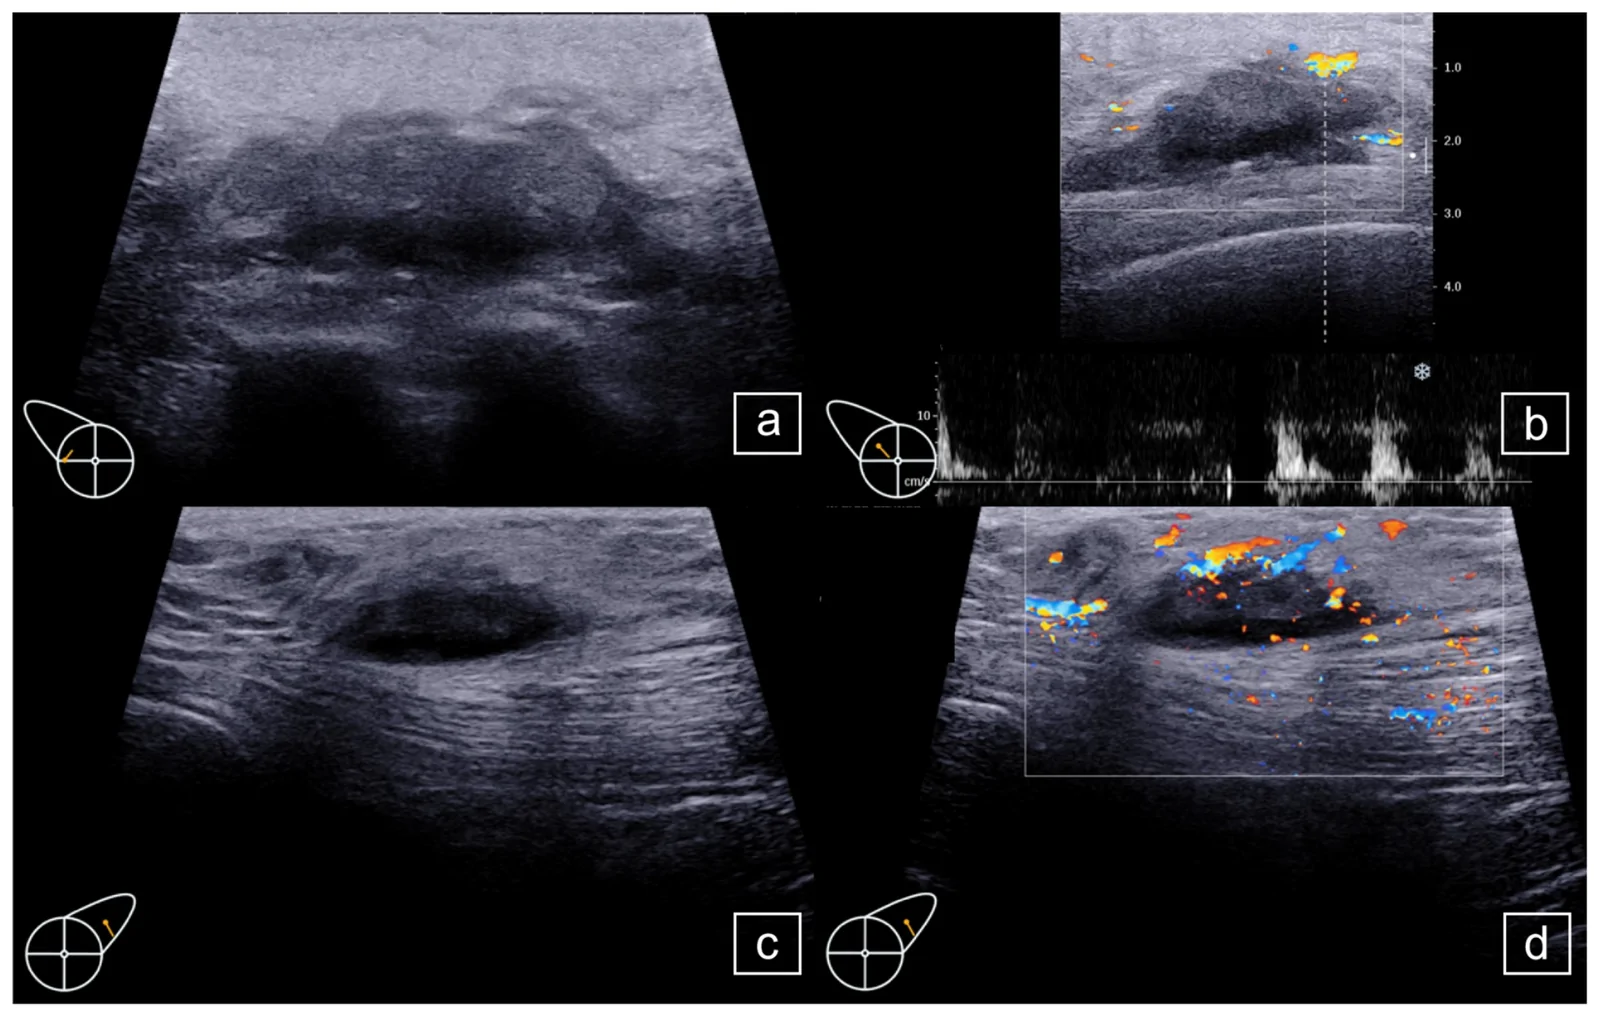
Ultrasound findings of right breast and bilateral axillary involvement. (a,b) Grayscale and color Doppler ultrasound of the lateral right breast extending to the axilla showing contiguous infiltrative hypoechoic masses with indistinct margins, irregular morphology, patchy internal hyperechoic areas, and internal and peripheral vascularity. (c,d) Grayscale and color Doppler ultrasound of the left axilla showing an irregular hypoechoic mass with indistinct margins and internal and peripheral vascularity. The white rectangular boxes in panels (b,d) indicate the color Doppler regions of interest, while the dashed line in panel (b) represents the Doppler sampling line used to obtain the spectral waveform.
